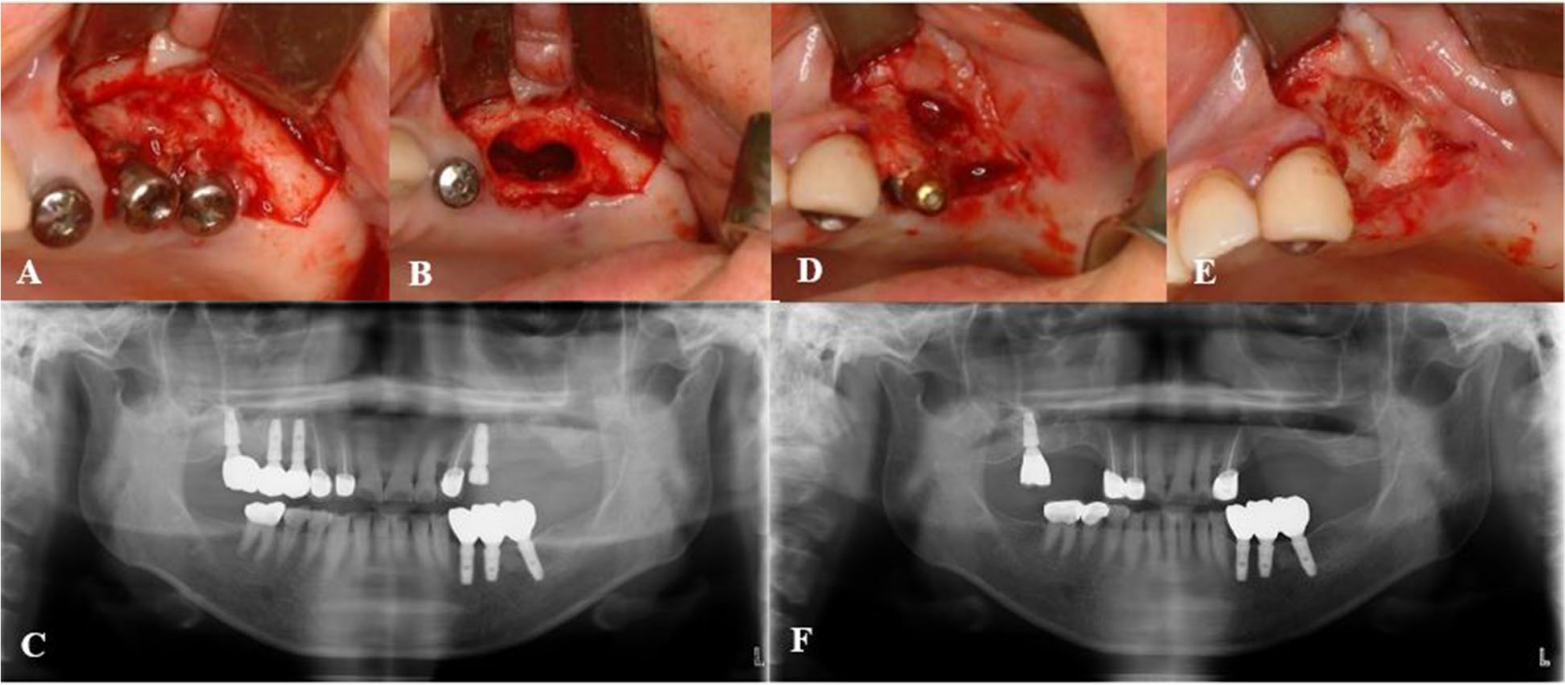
Surgical treatment of medication-related osteonecrosis of the jaw in breast cancer patient. A Inflammation at #26, 27 implant area. B Implant removal and saucerization at #26, 27 area. C Postoperative panoramic radiograph after 3 days showing clean resection of the lesion. D Inflammation at #24 implant area after 1 year and 2 months after the initial surgery. E Implant removal and saucerization performed. F Postoperative panoramic radiograph after 5 years and 3 months showing stable results at both surgical sites
